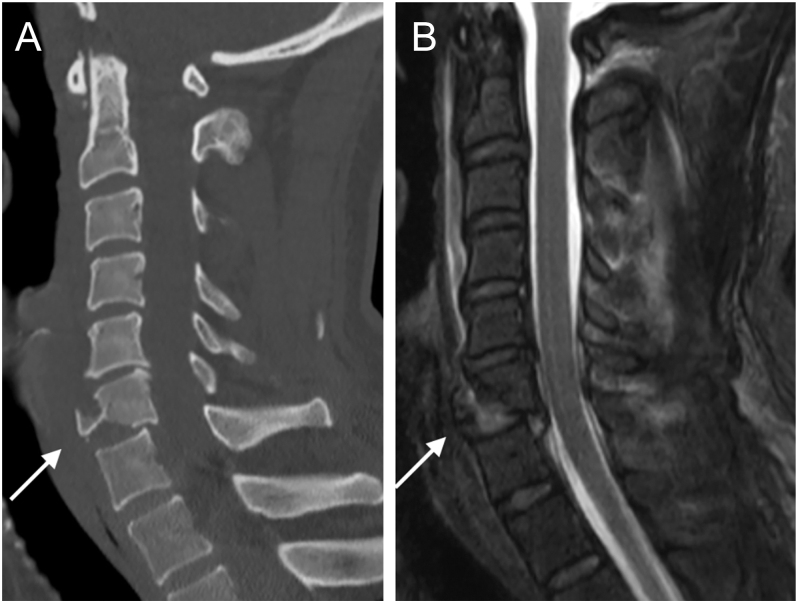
CT and MRI study of a C6 teardrop fracture. An anteroinferior fragment is observed, connected to the caudal VB by the disc and ALL (arrow). Notice the retrolisthesis of the larger C6 VB fragment, disc disruption, and spinal cord signal changes.
